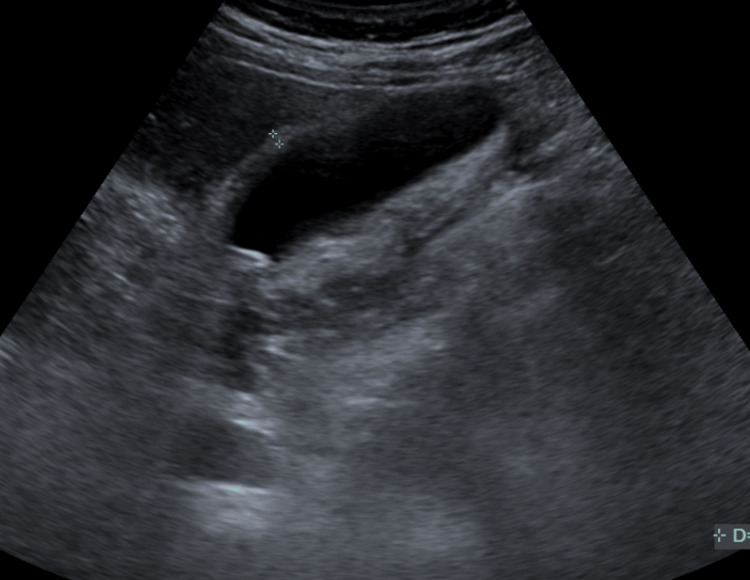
Ultrasound of the gallbladder and common bile duct with no common bile duct dilatation
